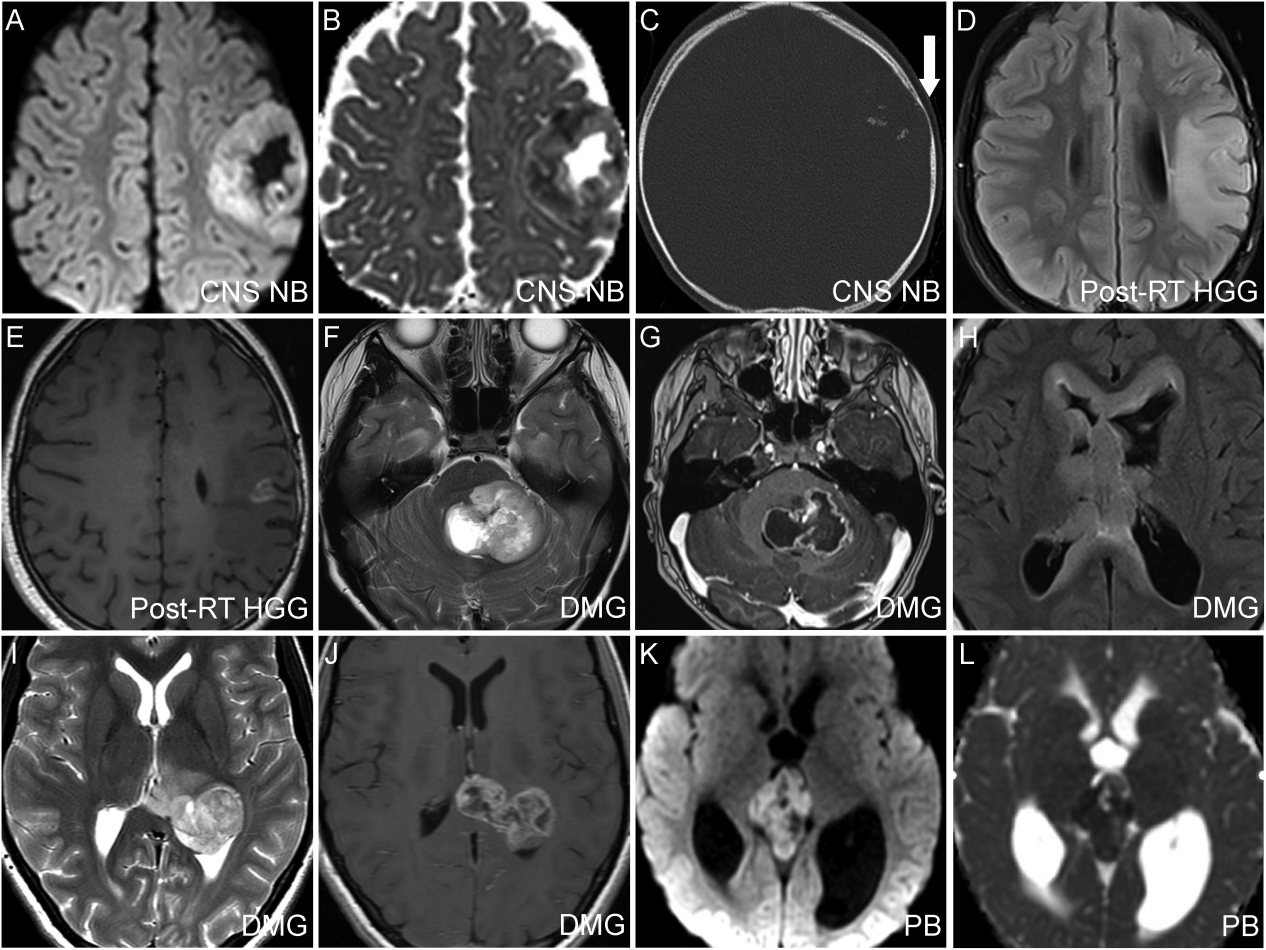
Imaging features of CNS tumors with *FOXR2* overexpression. Baseline axial diffusion-weighted (A), Apparent Diffusion Coefficient (ADC) map (B), and CT (C) images showing a hypercellular left frontal CNS NB-FOXR2 with coarse calcifications and cortical remodeling, suggestive of a CNS NB-FOXR2 (C, arrow). Axial FLAIR (D) and post-contrast T1 (E) images showing an infiltrative left frontal high-grade glioma (HGG) with enhancement several years after radiation therapy for a CNS NB-FOXR2 at the same location. Axial T2 (F), and post-contrast T1 (G) images showing a heterogeneously enhancing left pontine diffuse midline glioma (DMG). (H) Four months post-RT fluid-attenuated inversion recovery (FLAIR) image showing extensive new subependymal metastases. (I) Axial T2 and post-contrast T1 (J) images showing a circumscribed and heterogeneously enhancing left dorsal thalamic DMG. (K) Baseline diffusion-weighted images and (L) ADC map showing a hypercellular pineal mass, suggestive of a pineoblastoma.
